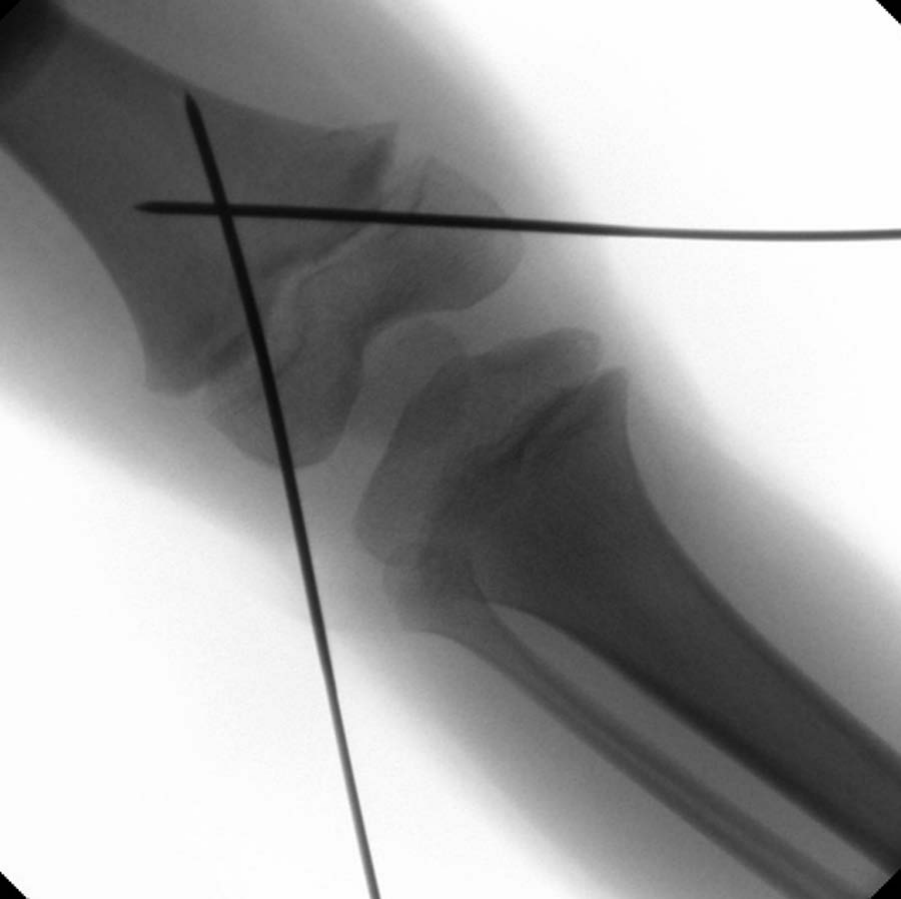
AP radiograph of the distal femur with satisfactory reduction and fixation with Kirschner wires.
